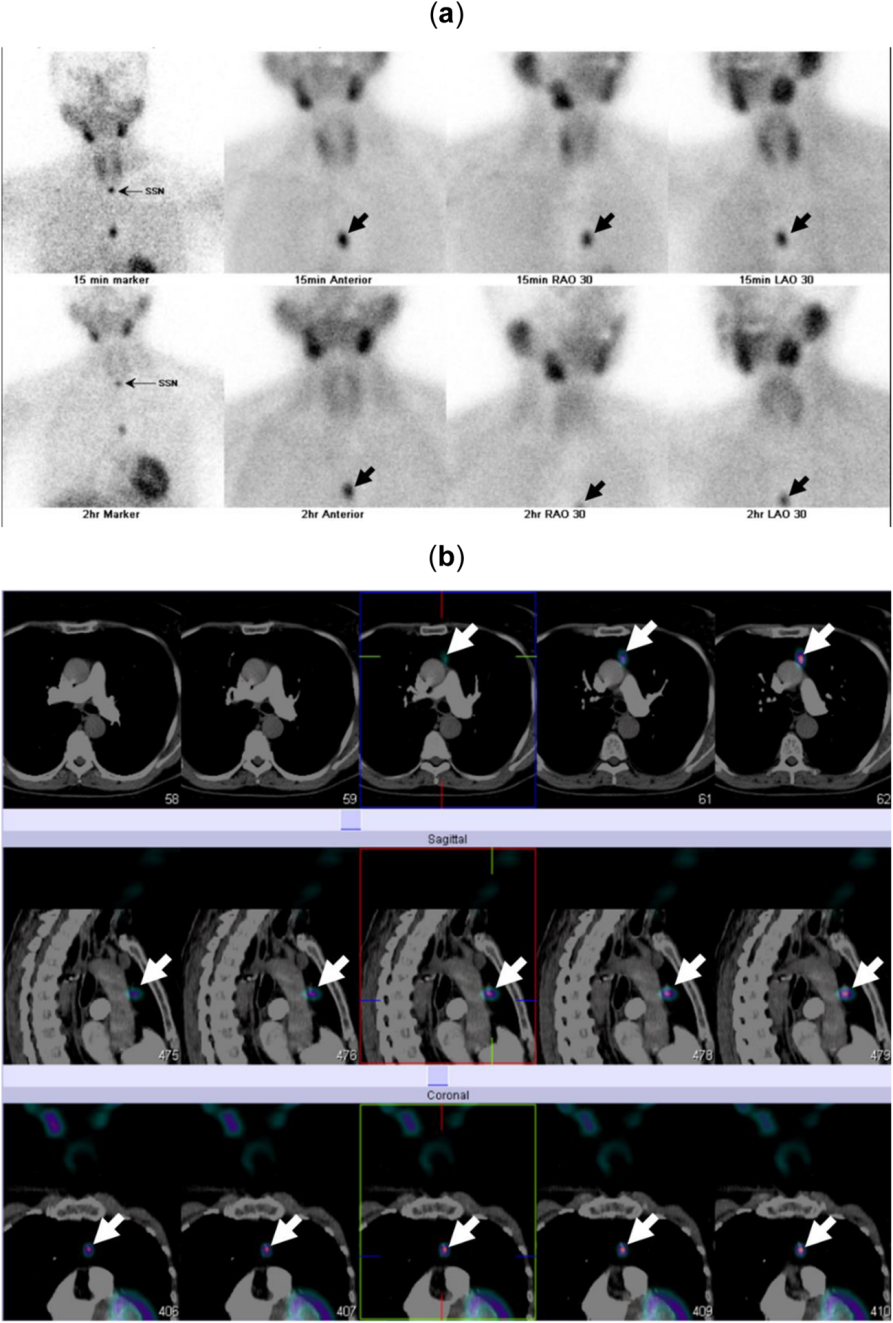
Anterior maximum intensity projection images from early (15 min) and delayed (2 h) phases of SPECT (a) and delayed-phase coronal, sagittal and axial fused ^99m^Tc-Sestambi-SPECT/CT images (b) in a woman (case 4) showing ectopic parathyroid adenoma in the anterior mediastinal fat sited just behind the sternum at level of the pulmonary trunk bifurcation
